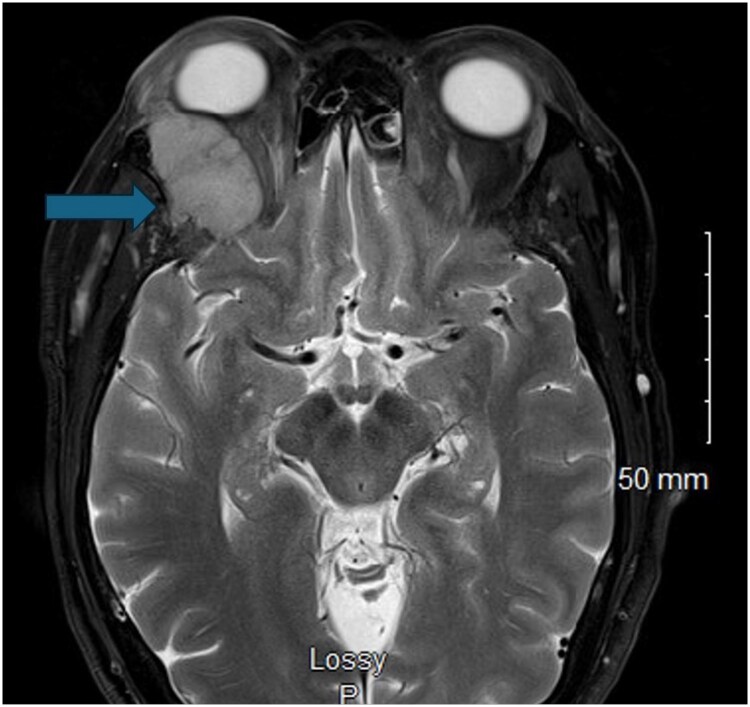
Magnetic resonance imaging of the orbit showing right lateral orbital wall mass lesion with effect on the adjacent optic nerve and increase in the right globe proptosis with increased displacement of the superior and lateral rectus muscles.
